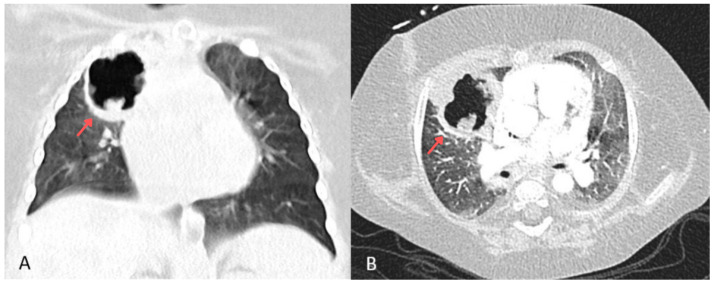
Contrast-enhanced chest CT, coronal section (A) and transverse section (B), performed 5.5 months after the initial examination. Arrows demonstrate a cavitary lesion in the right upper lung lobe.
